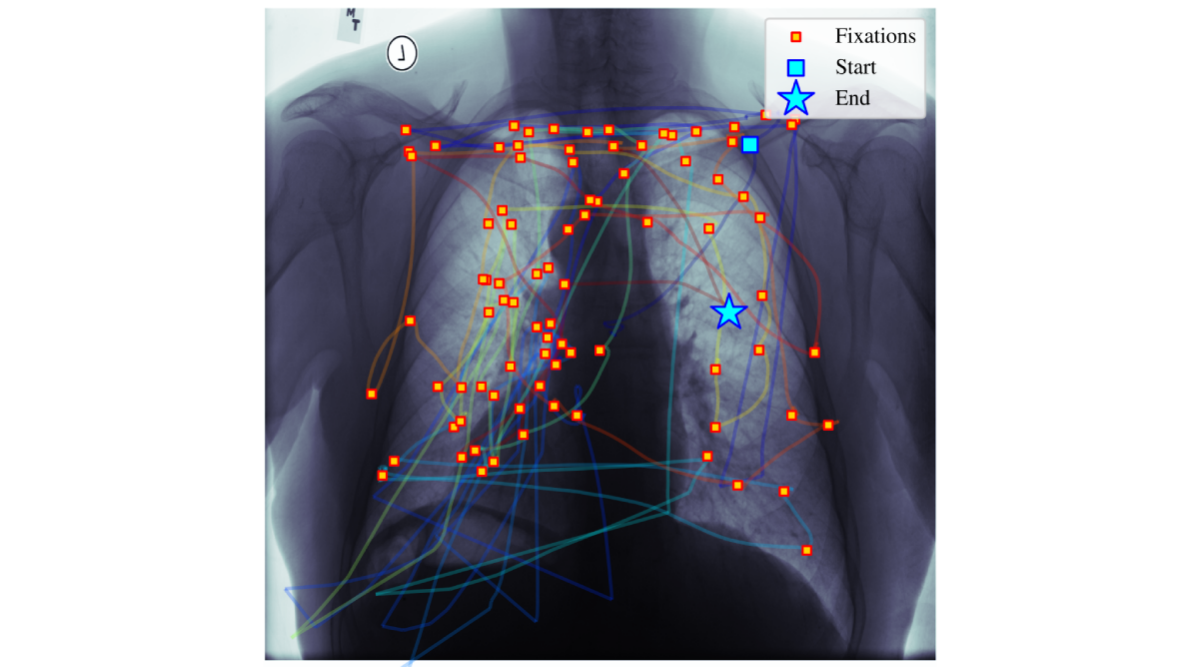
Scan of chest x-ray by participant 1 (faculty).
